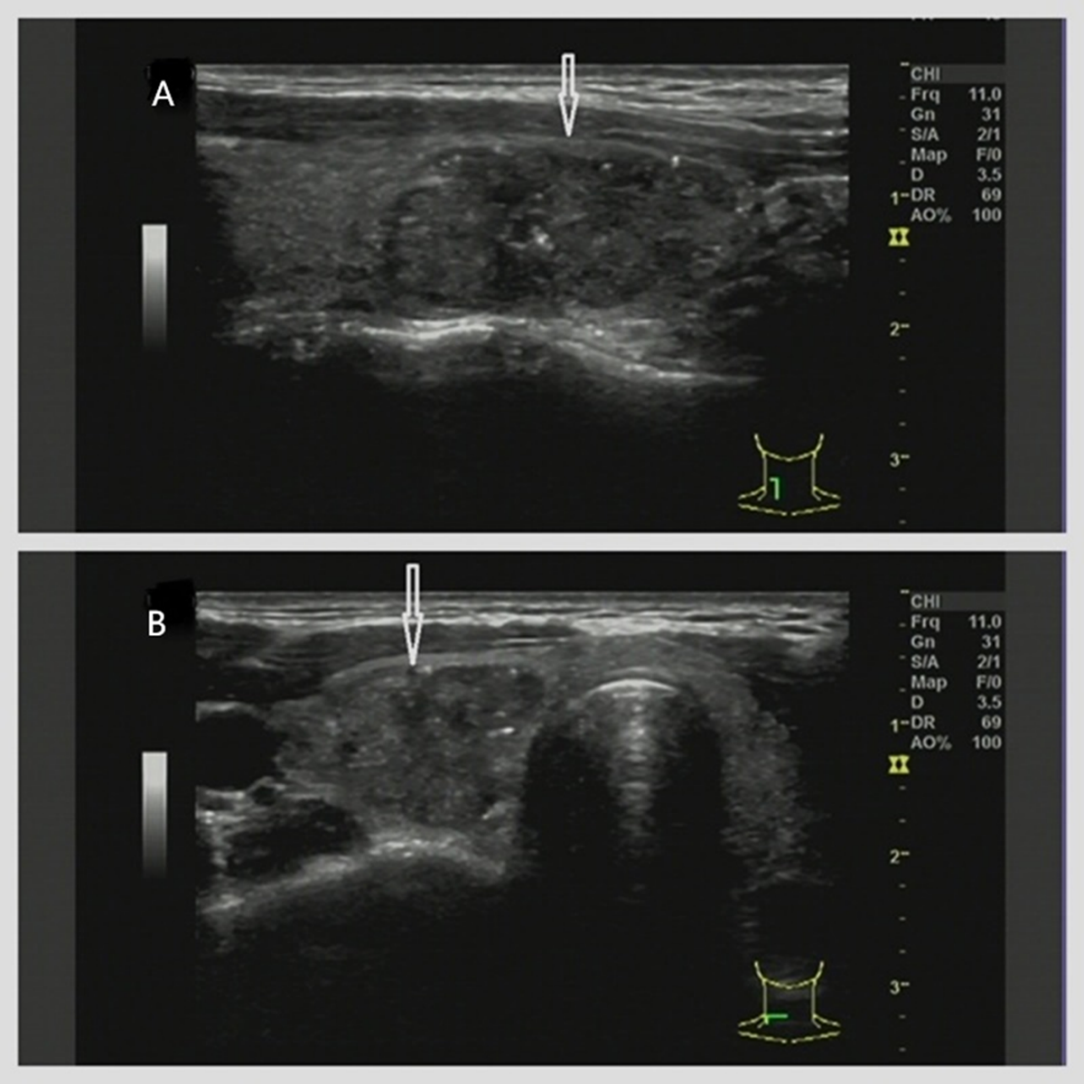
(A–B) A 29-year-old female with a pathologically confirmed thyroid nodule (papillary carcinoma). Ultrasound reveals a hypoechoic nodule (arrows indicate) in the right thyroid lobe with the following characteristics: irregular shape (no taller-than-wide), mixed solid-cystic composition, scattered punctate hyperechoic foci, comet-tail artifact, partially discernible margins, and dimensions of 27.7 × 19.1 × 13.0 mm. No posterior acoustic features observed. Classification: classical C-TIRADS 4b, modified C-TIRADS 4c, K-TIRADS 4.
